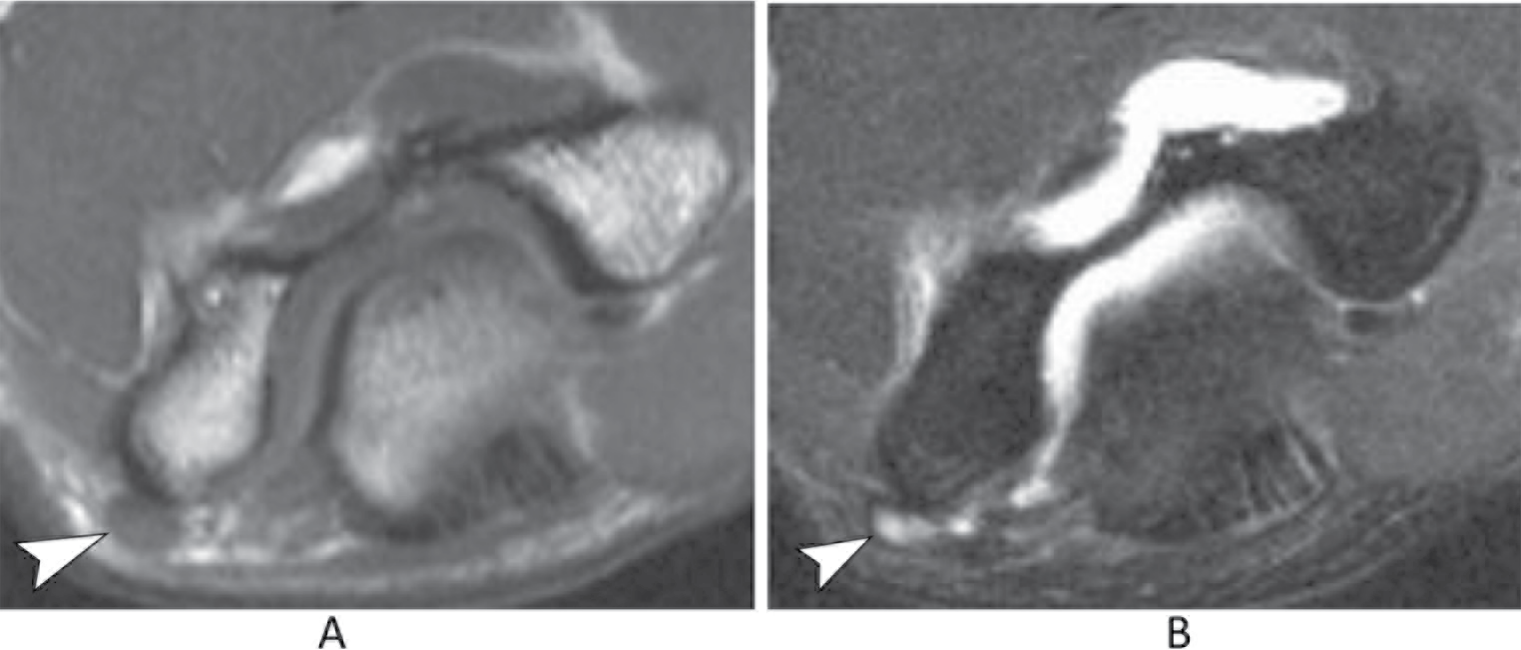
Ulnar nerve subluxation. Axial T1-weighted (A) and T2-weighted with fat suppression (B) images of the elbow demonstrate the ulnar nerve at the apex of the medial epicondyle (arrowheads), indicating subluxation. There is ulnar neuropathy associated, with increased signal and thickening of the nerve
